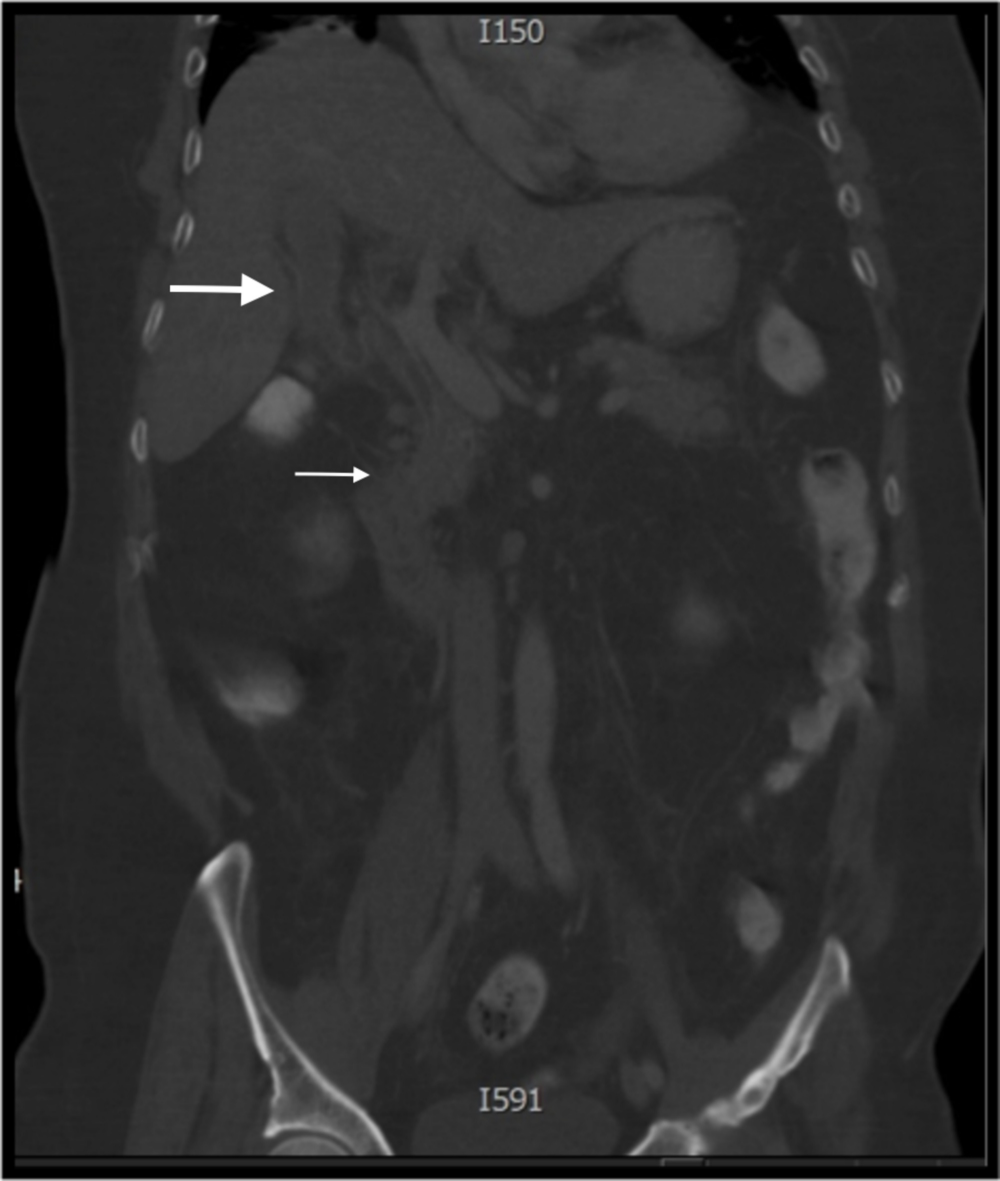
Abdominal computed tomography scan, coronal view Gallbladder distension with pericholecystic fluid (large white arrow) without evidence of gallstones or significant intrahepatic or extrahepatic common bile duct dilation or pancreatic duct dilation. Homogenous pancreatic enhancement and peripancreatic fat stranding, consistent with acute uncomplicated pancreatitis (small white arrow).
